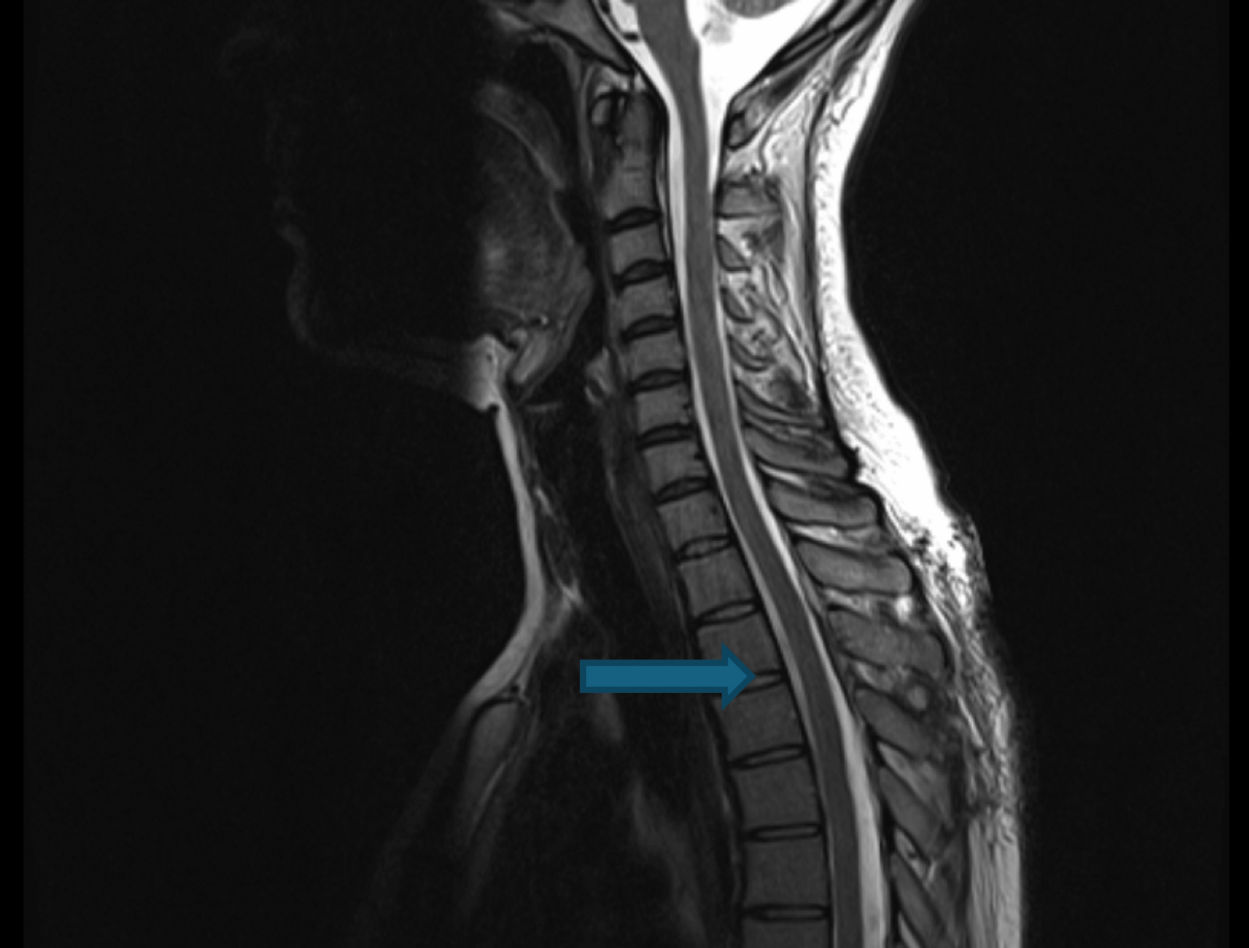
Sagittal T2-weighted MRI showing post-operative resolution of intrathecal haematoma at T3-T4. The blue arrow indicates the position of the resolved intrathecal haematoma on sagittal MRI.
